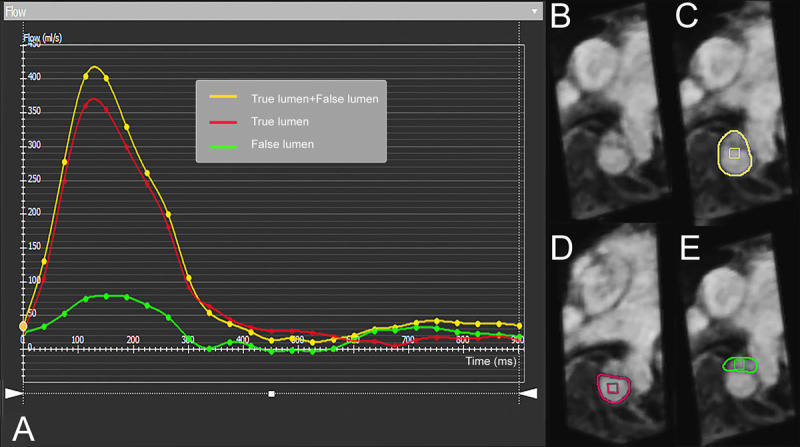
Quantitative flow evaluation of true lumen and false lumen using 4-dimensional (4D) flow magnetic resonance imaging (MRI). 4D flow MRI provides quantification of flow parameters depicted by the marked areas on panels B-E. The flow volume is displayed in a graph (A) where the y-axis represents the flow volume (mL/s) through the marked area and the x-axis represents time in milliseconds. In aortic dissection, the true lumen (A: red line, D: red outline) and the false lumen (A: green line, E: green outline) can be interrogated, and the entire flow volume (A: yellow line, C: yellow outline) can be determined.
